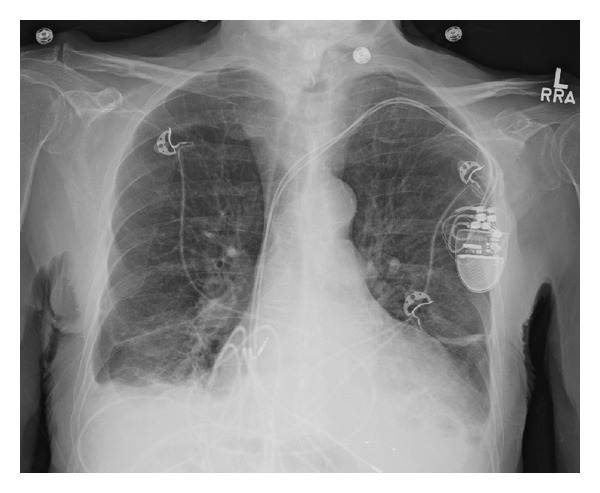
Chest X-ray demonstrating cardiomegaly, pleural effusions, and pulmonary vascular congestion.
